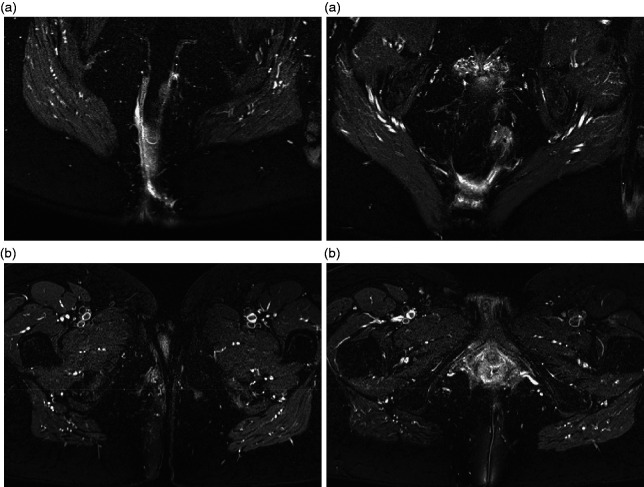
Magnetic resonance imaging of case 1 before (a) and 3 months after (b) HBO.
